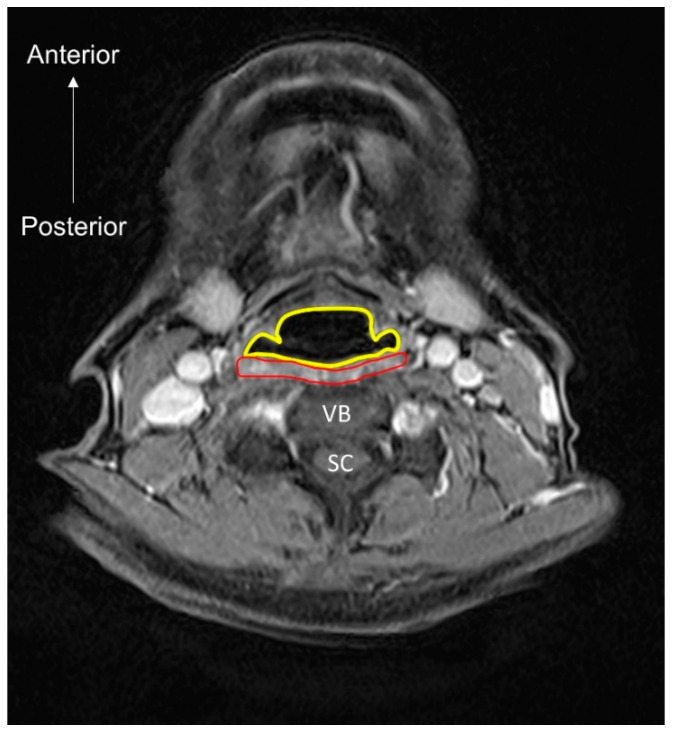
MRI (axial view) of the pharyngeal lumen (encircled by the yellow line) and muscle (encircled by the red line). VB, vertebral body; SC, spinal cord.
